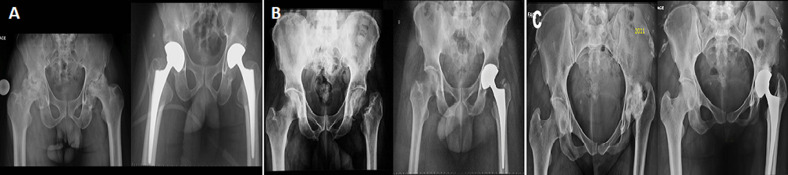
A) preoperative and postoperative AP pelvic radiographs demonstrating avascular necrosis in hemoglobinopathy; B) a femoral neck non-union; C) a childhood chronic arthritis sequalae
